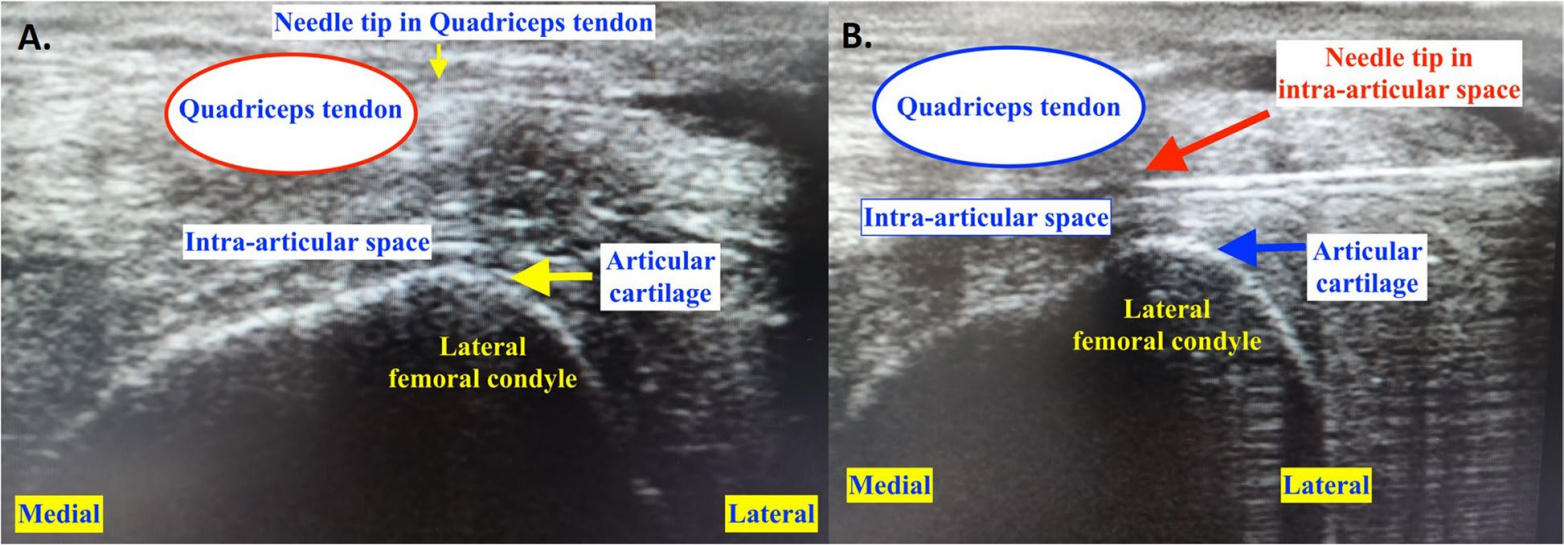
Ultrasonographic visualization of the needle tip to confirm extra-articular (A) and intra-articular (B) positions. Extra-articular position was defined as the needle tip was at the quadriceps tendon. For intra-articular position, the needle tip was in the intra-articular space beneath the quadriceps tendon and above the articular cartilage of lateral femoral condyle
